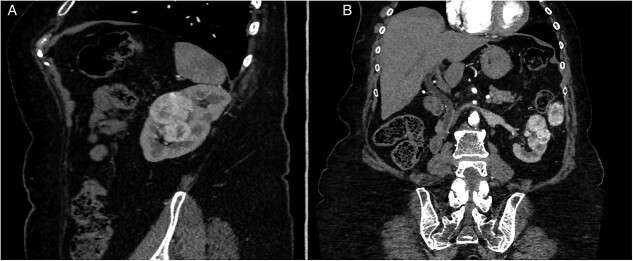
Preoperative CT-scan. (A) Sagittal section. (B) Modified coronal section allowing to see the left pericolic gutter metastasis.
